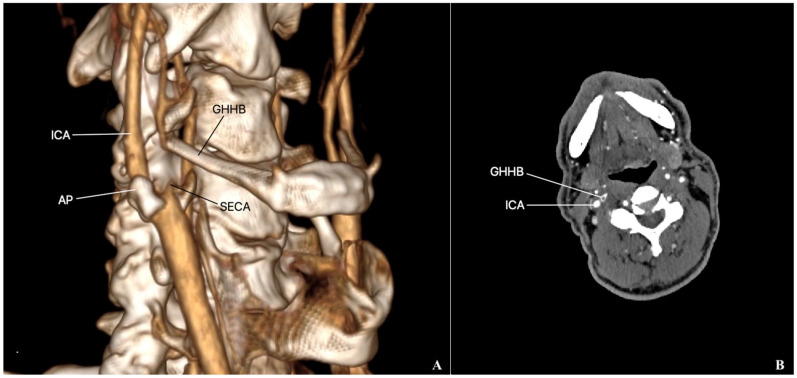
(A) CTA reveals the atherosclerotic stenosis of the right ICA and the entrapment of the right ECA by the GHHB. (B) Axial view of the carotid bifurcation. ICA: internal carotid artery; AP: atherosclerotic plaque; GHHB: greater horn of the hyoid bone; and SECA: stump of external carotid artery.
